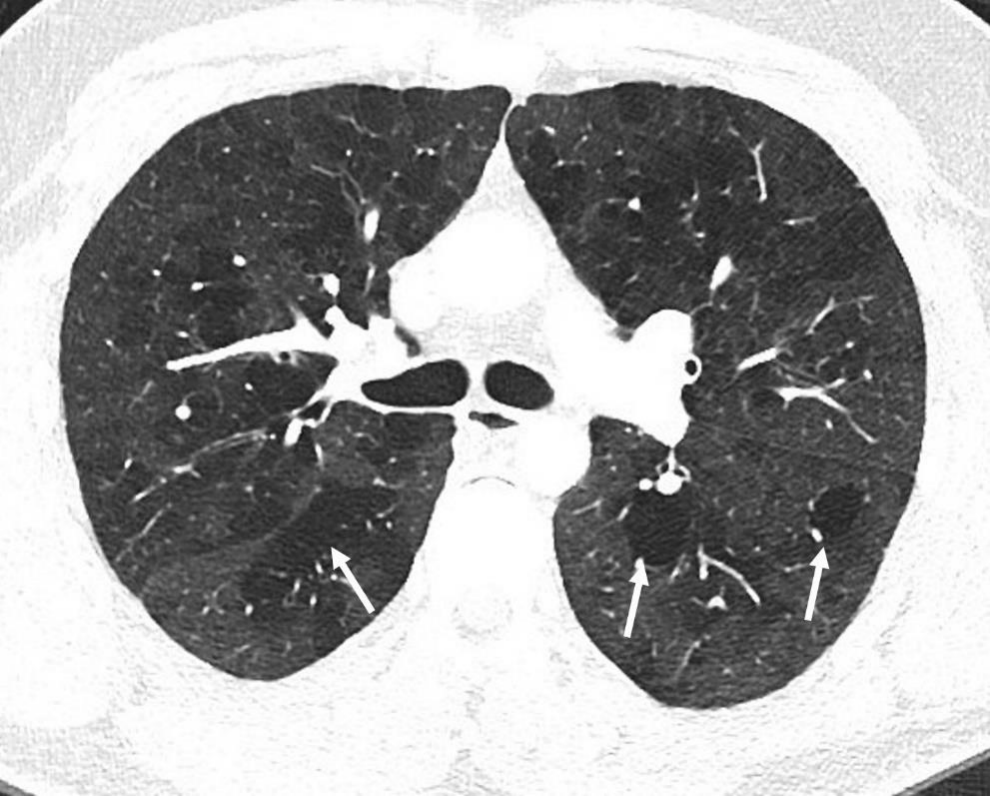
An axil CT image (lung window) of the chest of a 54 years old male smoker shows widespread emphysematous changes in both the lungs. These changes appear as poorly marginated lucent areas of varying sizes within the lung parenchyma (“holes in lung”). A few of these areas in bilateral lower lobes are marked (solid arrows). Right oblique fissure is not delineated on the image
